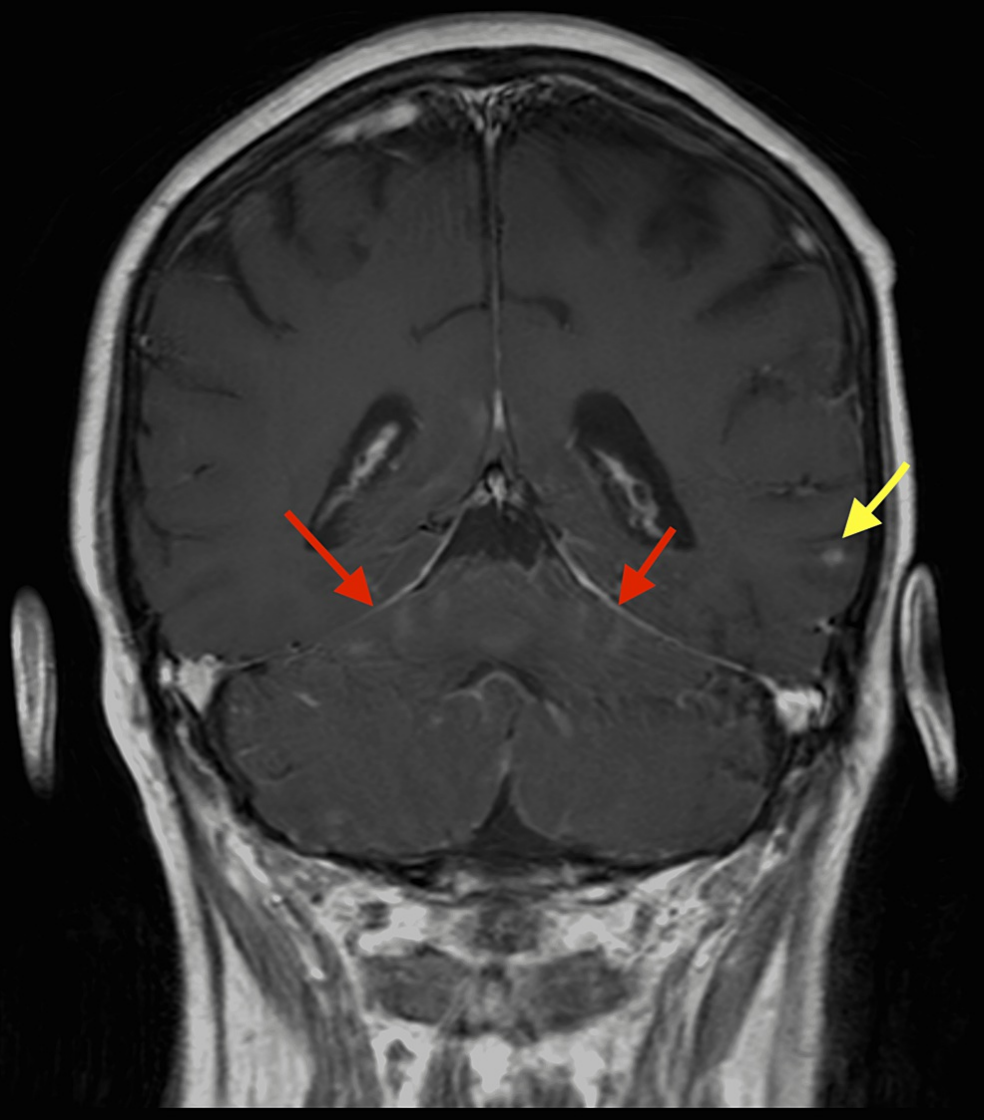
Brain MRI coronal view during T1 post-contrast showing interval leptomeningeal enhancement (red arrow) during hospitalization. The yellow arrow shows stable enhancing focus lateral left temporal lobe from previously treated metastatic disease. MRI: magnetic resonance imaging
